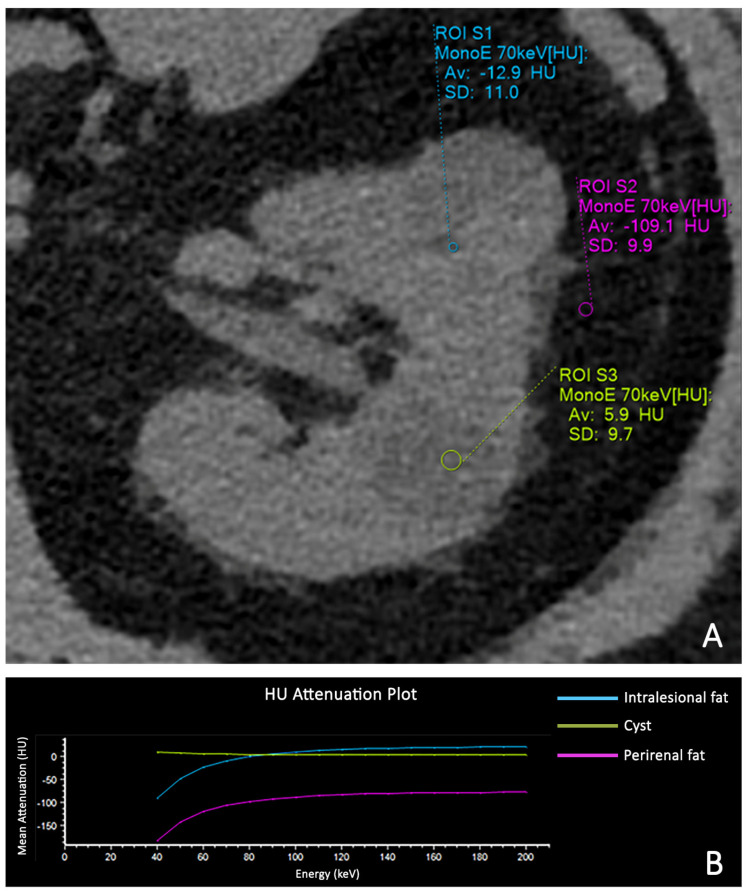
Unenhanced monochromatic 70 keV CT image showing a minimal amount of hypodense tissue in the rear part of the anterior mass with negative values (Hounsfield Unit −12.9). The other two ROIs are placed in the cyst (HU 5.9) and in the perirenal fat (HU −109.1) (A). HU attenuation curves derived from unenhanced monochromatic CT images from 40 to 220 keV. Both intratumoral hypodense foci (blue line) and perirenal fat (purple line) exhibit a decrease in attenuation as energy is reduced, which is characteristic of adipose tissue. No difference in curve attenuation is visible for a cystic mass (green line) (B).
